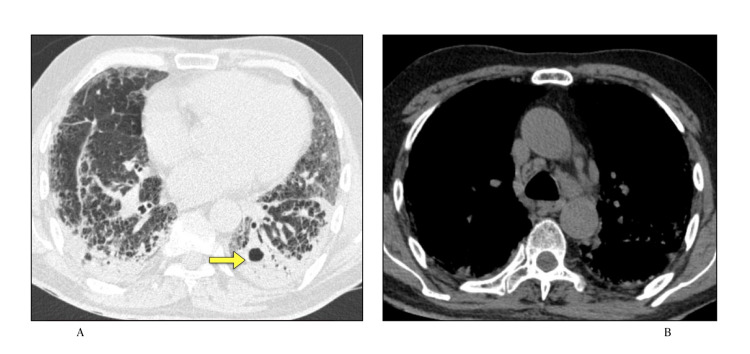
HRCT Chest (lung and mediastinal windows) A) Focal consolidation in left lower lobe with focal cystic changes (air bubble sign- shown by arrow) with background changes of fibrosis B) Enlarged mediastinal lymph nodes
